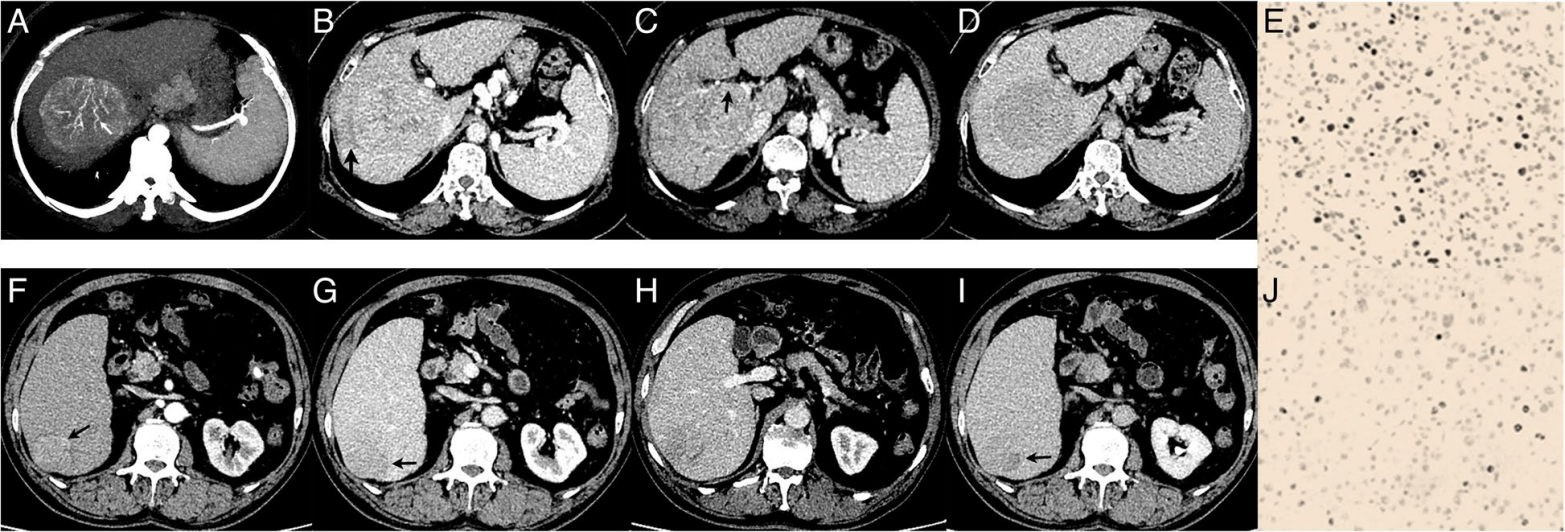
Representative images of multiphase contrast enhanced CT (CE-CT). A-E high Ki-67 HCC lession (black arrow), A, non-rim APHE and internal arteries (white arrow) on AP, B-C, hypodense halo and PVTT on PP, wash out and enhancing capsule on DP; consisting of “internal arteries” and “hypodense halos” defined as TTPVI, E, High and low Ki67 expression (70.5%), the brown regions represent positive Ki67 expression; And F-J show low Ki-67 expression HCC lesion (black arrow), F, no internal arteries on AP, G-I, no hypodense halos and PVTT on PP, non-enhancing capsule on DP, no TTPVI present. J, low Ki67 expression (8.2%); AP, arterial phase; PP, portal venous phase; DP, delayed phase; PVTT, portal vein tumor thrombus; TTPVI, two-trait predictor of venous invasion
